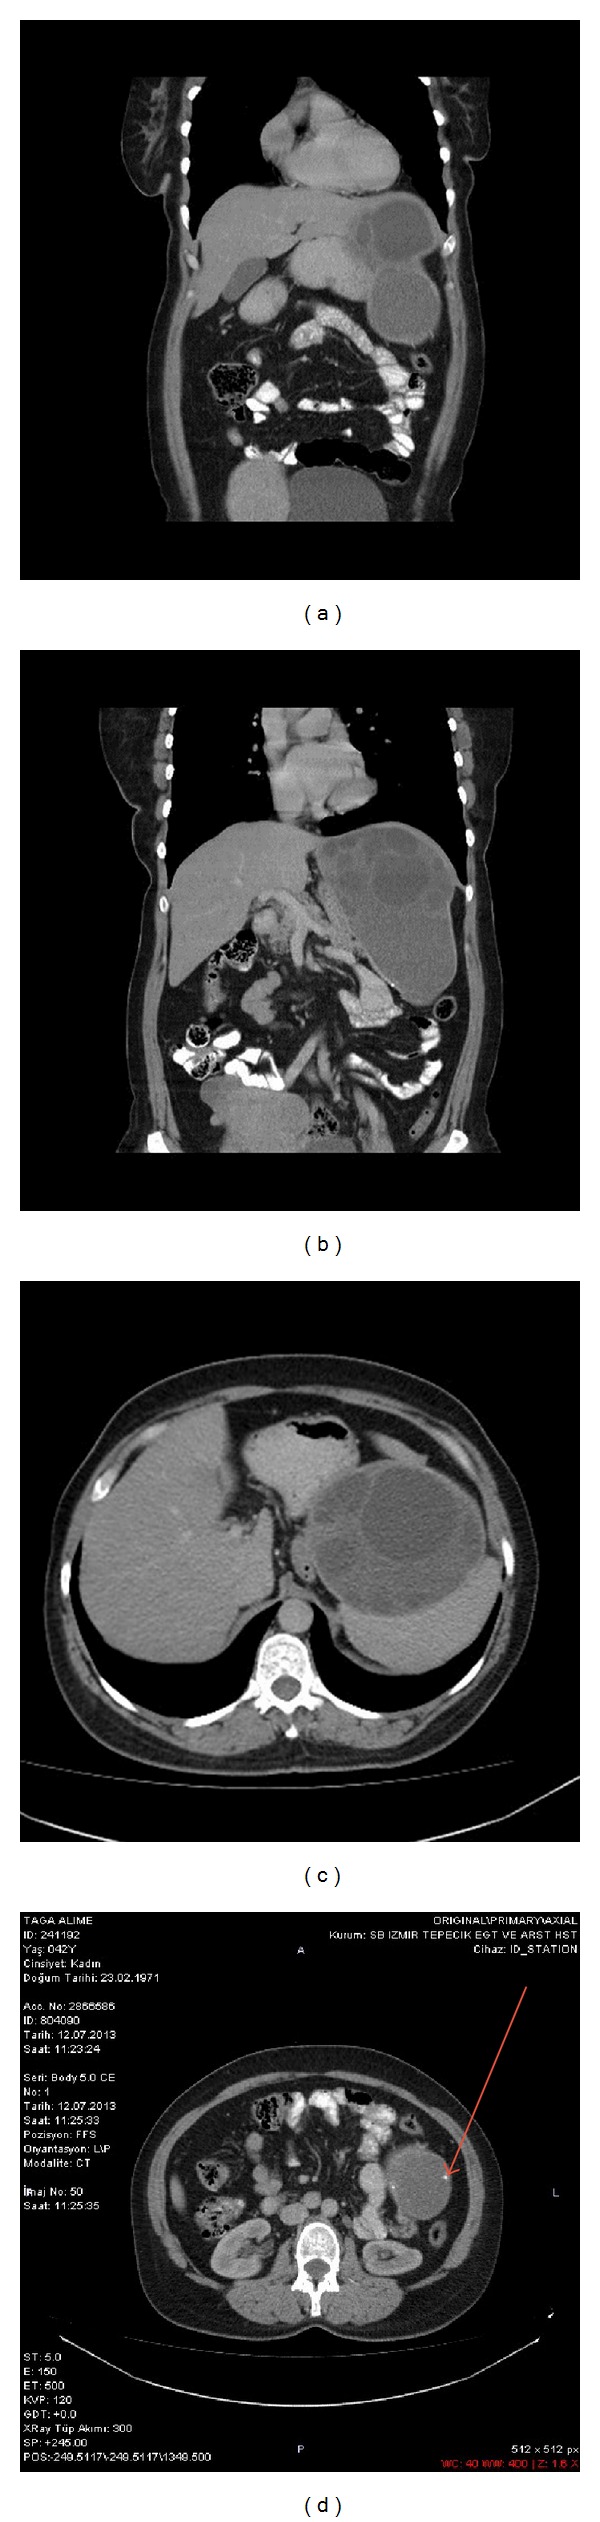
(a) The coronal CT image of bilobar hydatid cyst lesion. (b) The coronal CT image of multiple daughter cysts. (c) The axial CT image of multiple daughter cysts. (d) The axial CT image of inferior portion of the cyst that the red arrow shows the cystic wall calcification.
